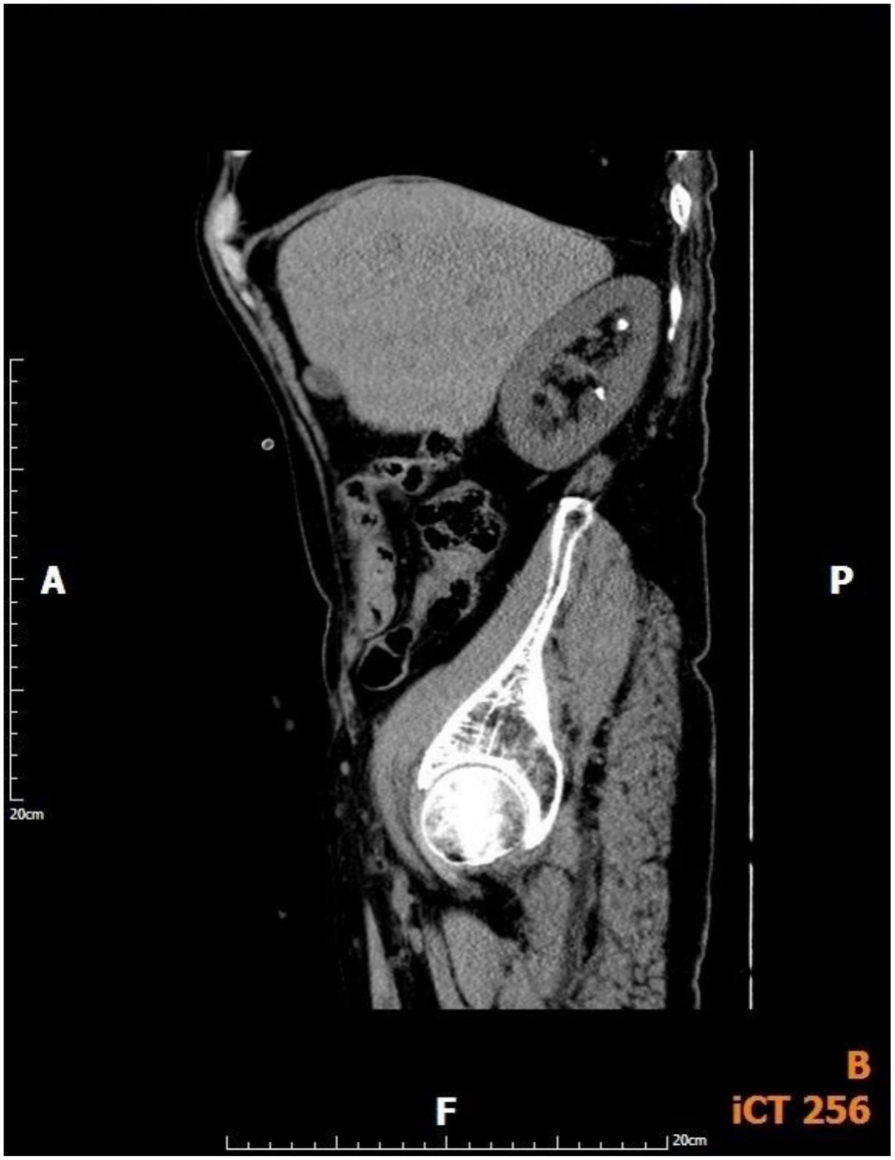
CT images of patient with incomplete stone removal on the first day after surgery.
